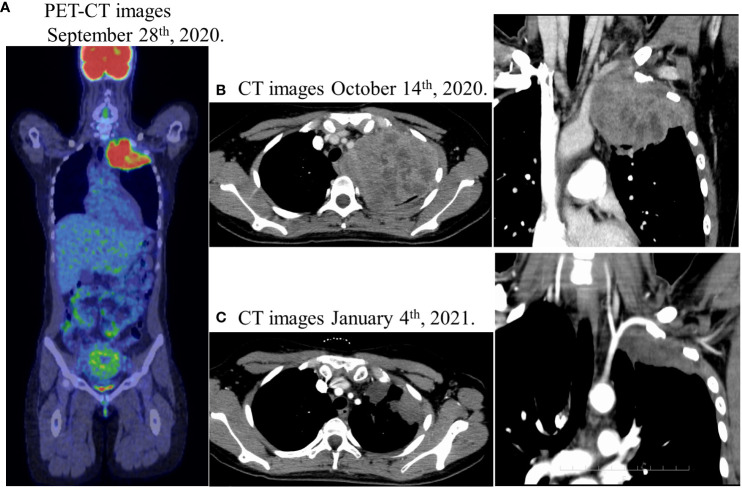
(A) Positron emission tomography-computed tomography (PET-CT) showed an abnormal FDG accumulation in the left pulmonary apex (SUVmax 12.58), no abnormal FDG accumulation in the gastrointestinal tract, and no suspicious findings of distant organ or lymph node metastasis. (B) Lung enhanced computed-tomography (CT) scan showed a massive soft tissue shadow, about 107 × 88 × 82 mm in the upper lobe of the left lung on October 14th. (C) Lung enhanced CT scan showed a soft tissue shadow, about 72 × 50 × 42 mm in the upper lobe of the left lung on January 4th after chemoradiotherapy, indicating a partial response with a reduction of 40%.
